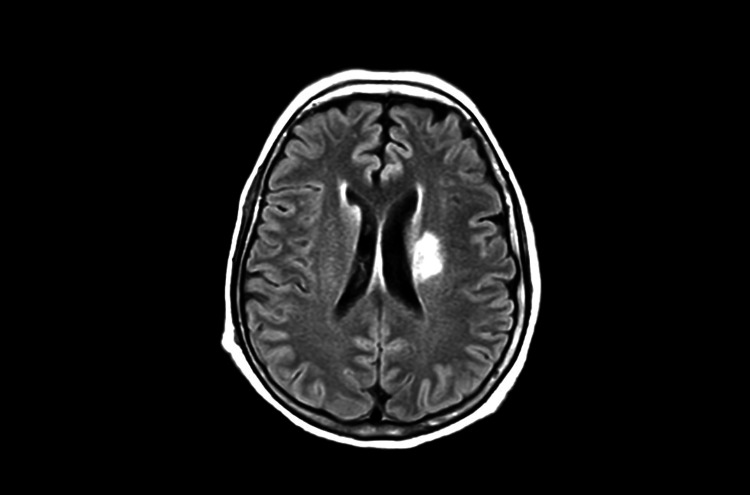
Axial FLAIR brain MRI showing focal hyperintense lesions in the left periventricular white matter compatible with ischemic changes involving deep cerebral structures FLAIR: fluid-attenuated inversion recovery, MRI: magnetic resonance imaging
